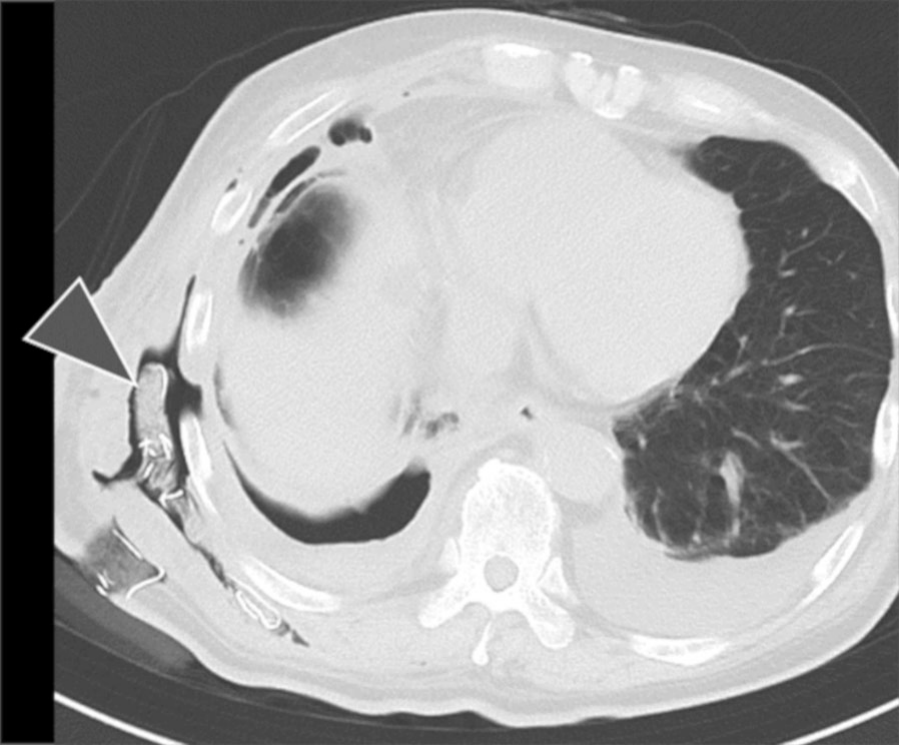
CT scan demonstrating bilateral pleural effusion and abscess cavity extending below the serratus anterior muscle. CT: computed tomography; Black triangle: abscess cavity
